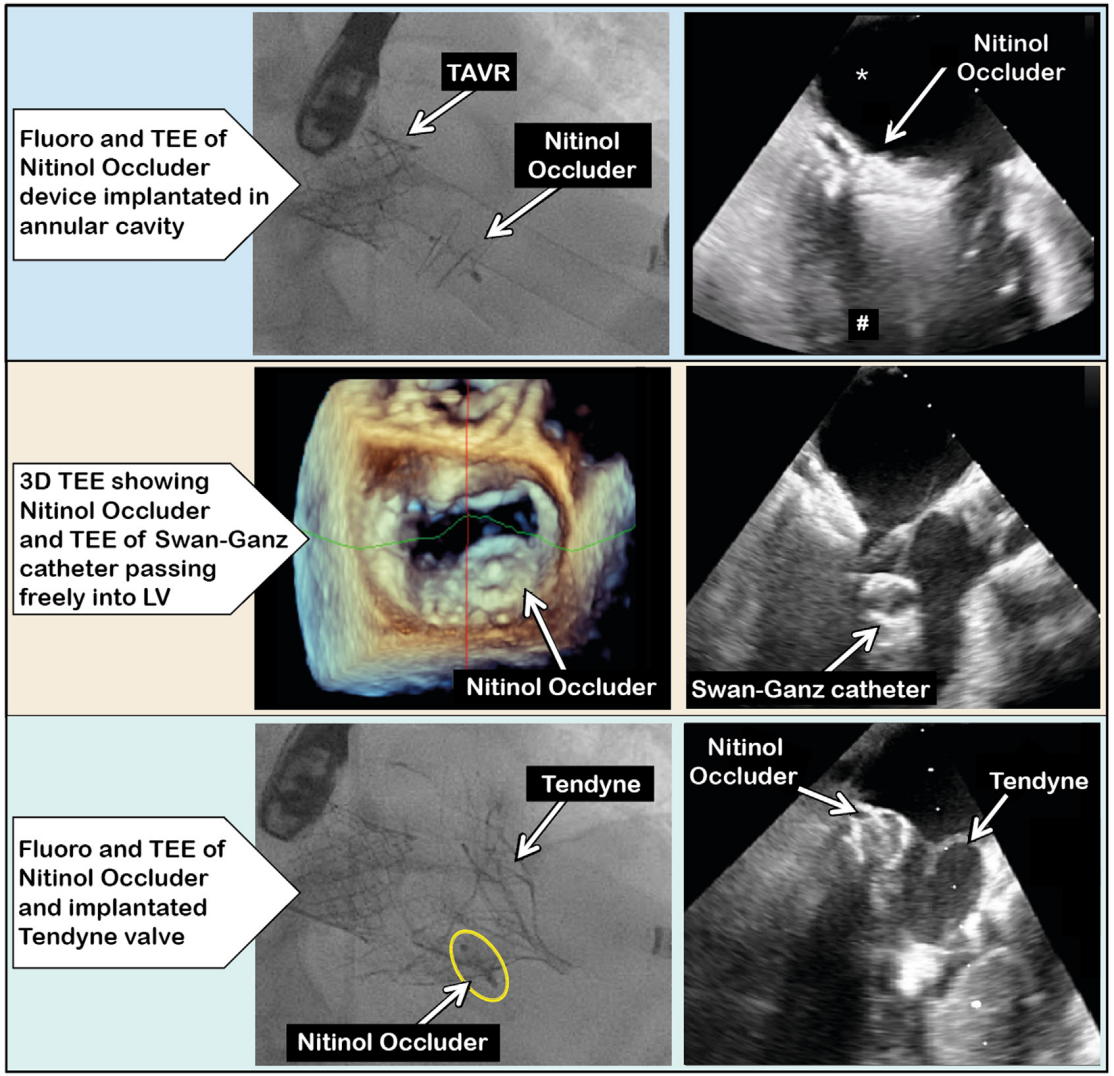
Combined Occluder Device and Transcatheter Mitral Valve Implantation Procedure Asterisk denotes inflow in left atrium and # outflow in left ventricle. LV = left ventricle; TAVR = transcatheter aortic valve replacement; TEE = transesophageal echocardiography.
